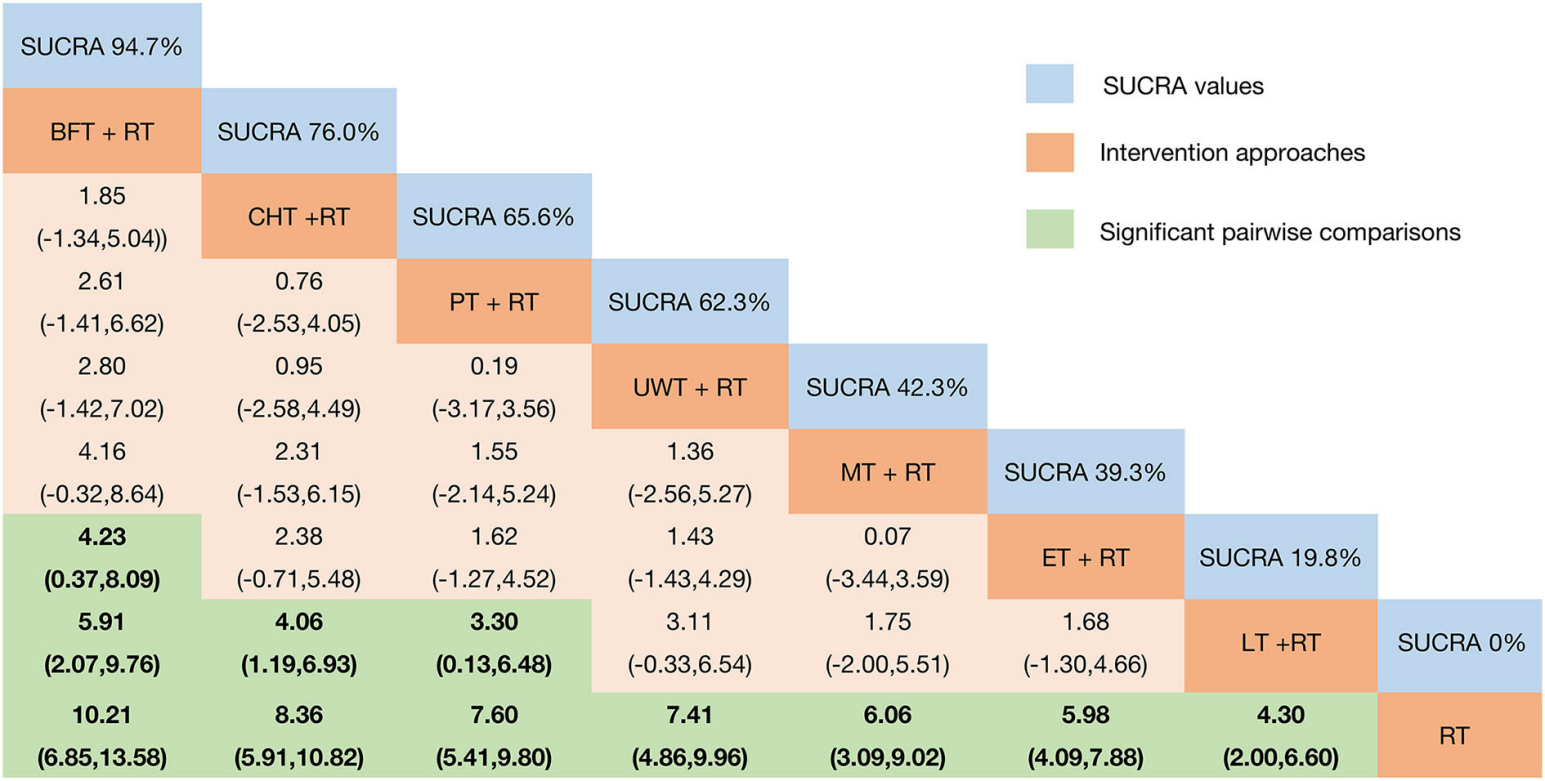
Relative effect sizes of FMA-UE efficacy after the intervention according to network meta-analysis. Treatments were ranked in order of their likelihood of being the best treatment. The numbers in the blue boxes are SUCRA values, representing the rank of the treatments. Meaningful pairwise comparisons are highlighted in green and bold. RT, rehabilitation training; ET, electrotherapy; LT, light therapy; UWT, ultrasonic wave therapy; CHT, conduction heat therapy; PT, pressure therapy; MT, magnetic therapy; BFT, biofeedback therapy.
